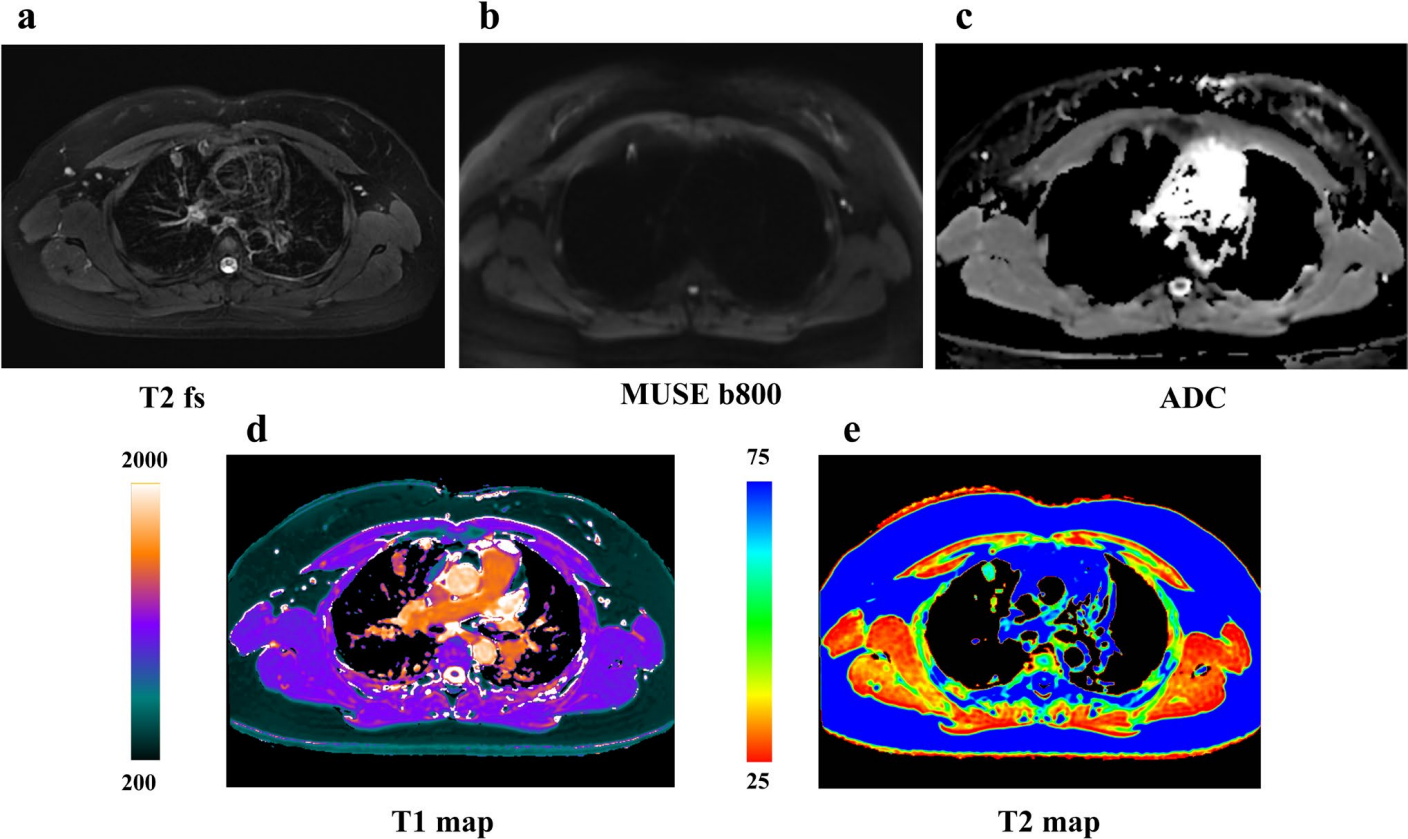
Female, 31 years old, solid nodule in the upper lobe of the right lung, approximately 15.6 mm in size, pathologically confirmed invasive lung adenocarcinoma with alveolar and papillary growths. (a) T2WI; (b) MUSE DWI (*b* = 800 s/mm^2^); (c) MUSE ADC; (d) T1 mapping (ms); and (e) T2 mapping (ms).
